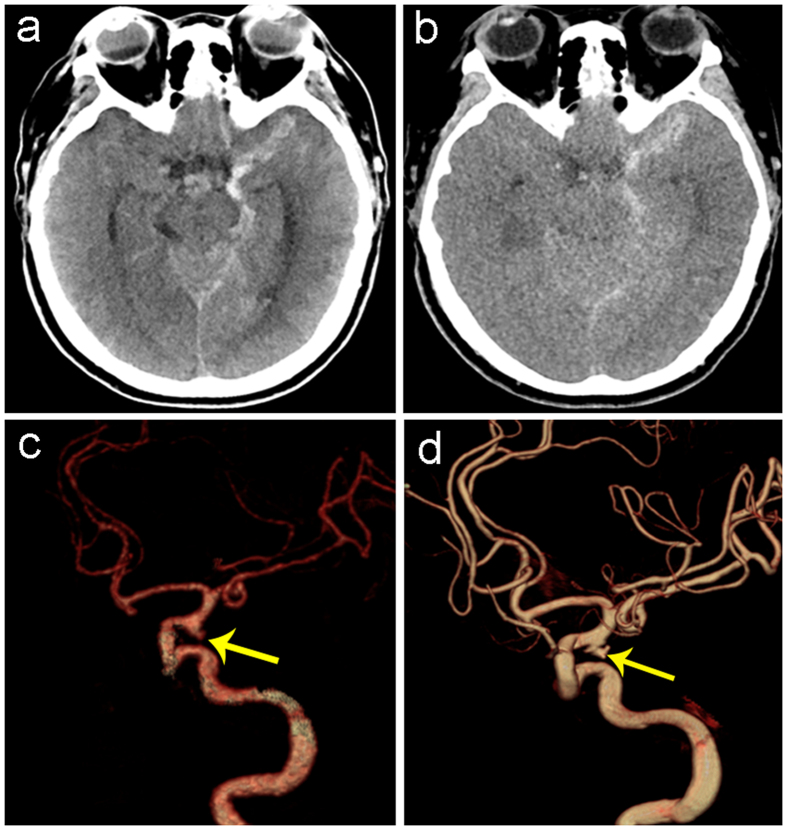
A 59-year-old women with spontaneous subarachnoid hemorrhage caused by ruptured aneurysm in left posterior communicating artery. (a) True non-enhanced CT image and (b) virtual non-enhanced CT image show subarachnoid hemorrhage, (c) volume-rendered dual-energy CTA image shows a true-positive aneurysm in the left posterior communicating artery (yellow arrow), which was confirmed by 3D-DSA (d).
